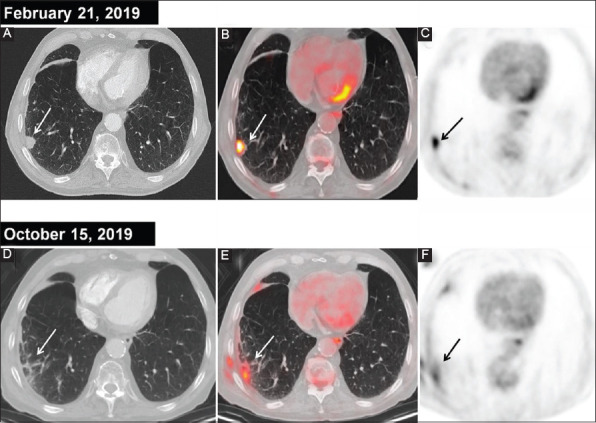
A 84-year-old male, smoker, with severe COPD, domiciliary oxygen therapy, and ischemic cardiomyopathy. He was diagnosed with a Stage I adenocarcinoma of the lung in the lower right lobe which was treated with stereotactic body radiation therapy (SBRT). The 18F-fluorodeoxyglucose (18F-FDG) positron emission tomography/computed tomography (PET/CT) images prior to SBRT (A, B, and C) showed the subpleural tumor in the right lower lobe with FDG uptake and SUVmax 8.2 (arrows). Six months after the end of SBRT, 18F-FDG PET/CT images (D, E, and F) show a ground- glass opacity and subsegmental atelectasis at the anterior lung node location (arrows).
